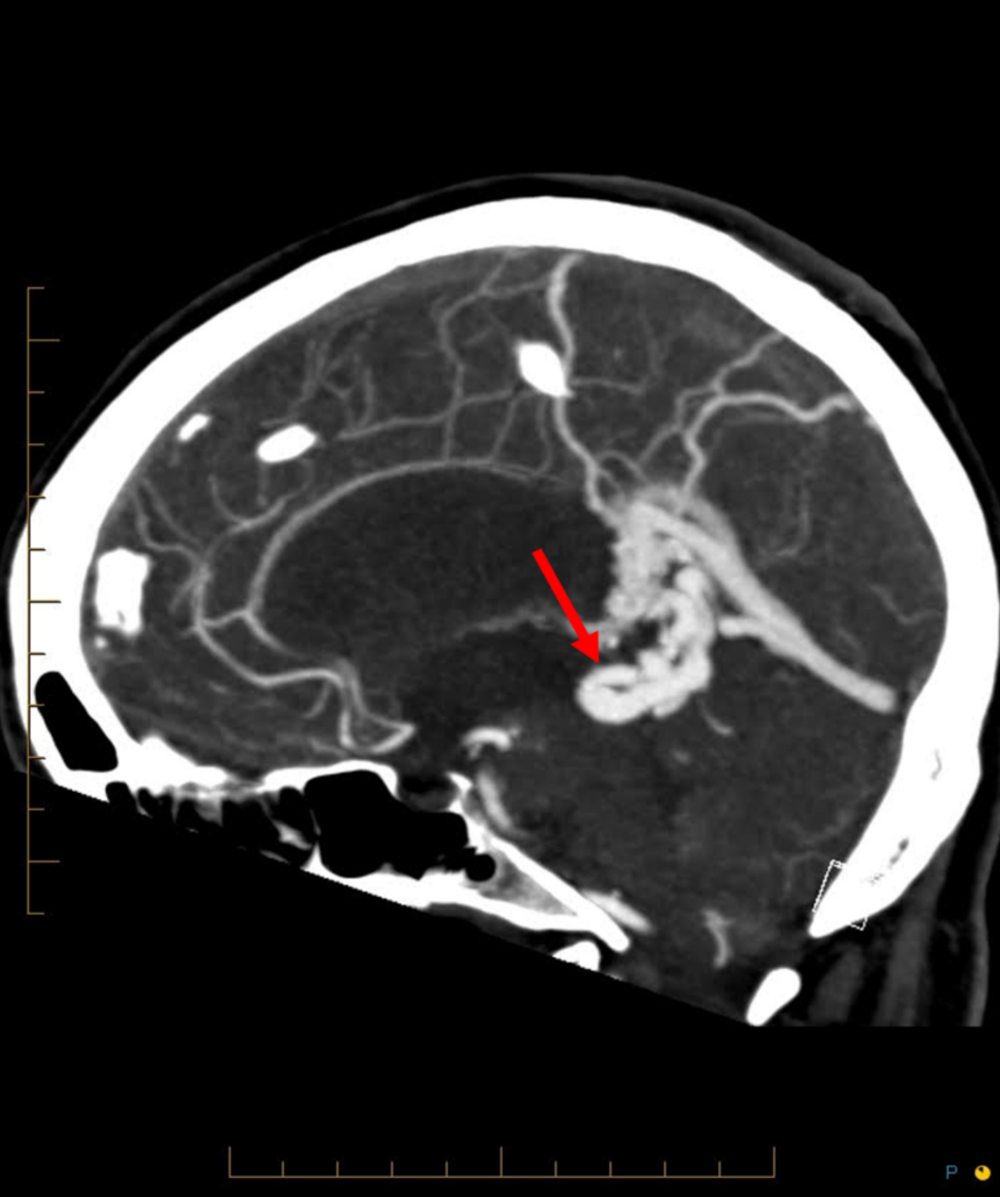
Sagittal computed tomography image of the brain The red arrow denotes where the arteriovenous malformation compresses the cerebral aqueduct.
